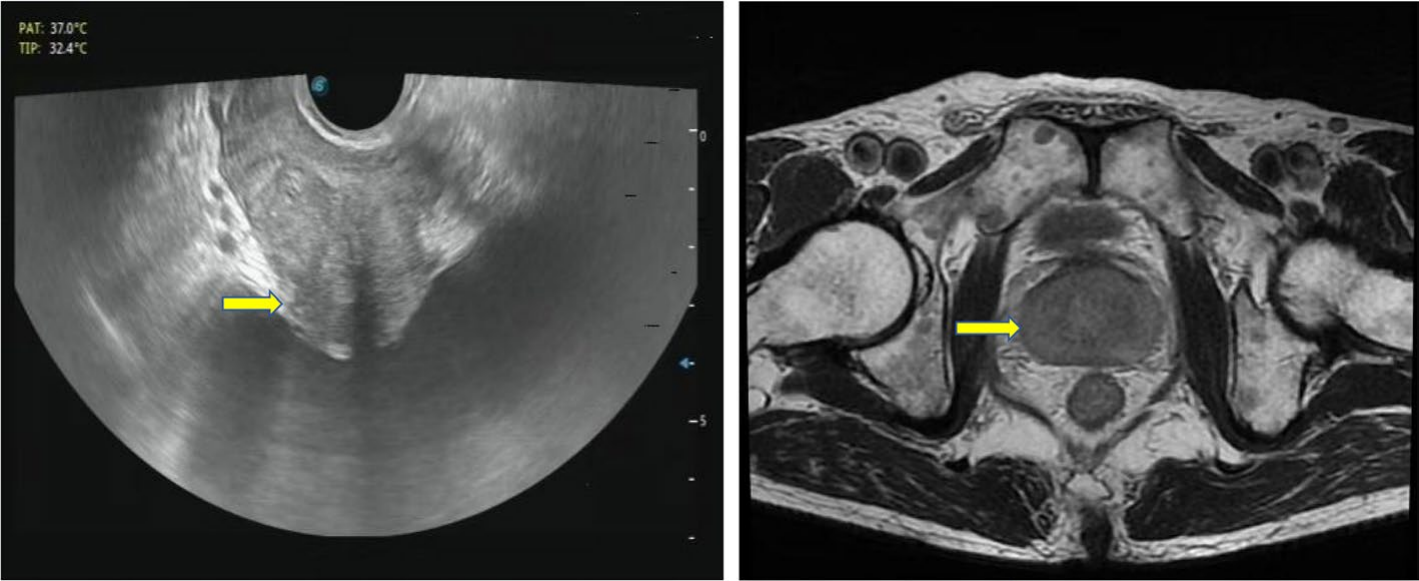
Prostate color ultrasound and magnetic resonance imaging (MRI) showed grade II prostate hyperplasia
